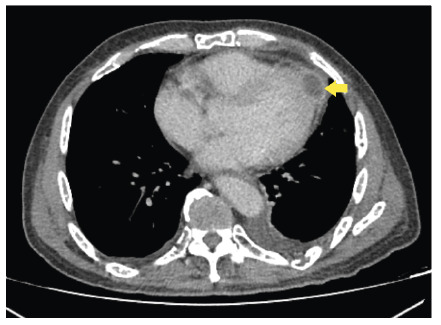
Computed tomography of the thorax showing a pericardial rim-enhancing collection measuring 2.6 x 1.9 x 1.6 cm.
